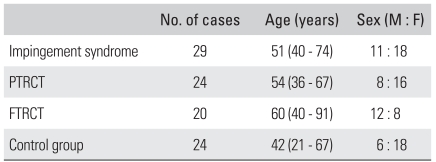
Demographic Findings in Quantitative SPECT Study
